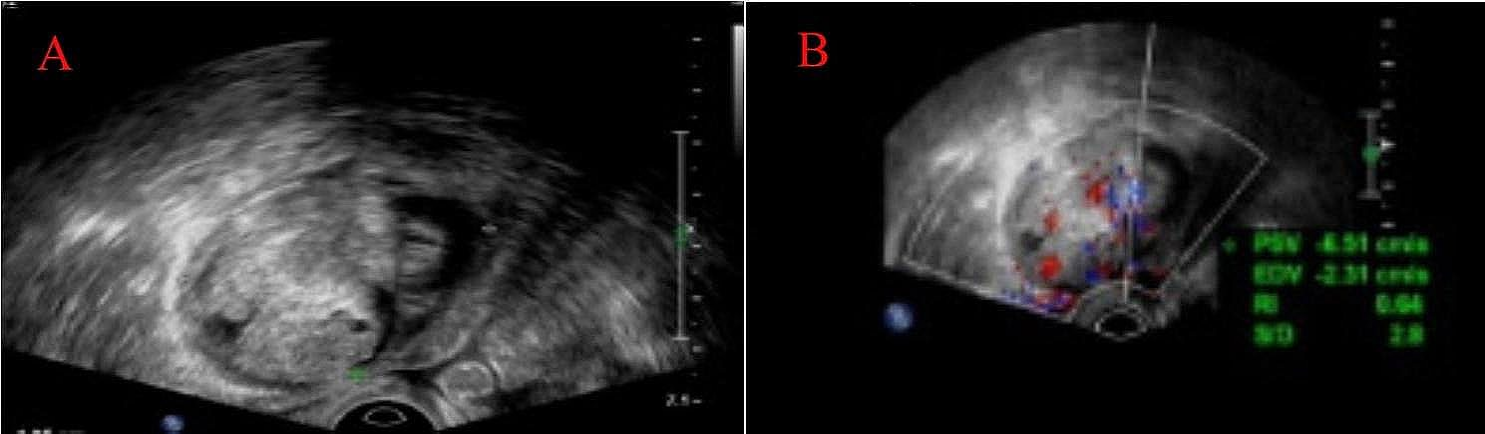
B-ultrasound image. Solid mixed pelvic echo area of 5.0 cm × 3.8 cm × 4.7 cm, with a clear boundary and regular morphology (A). CDFI: Rich blood flow signals can be detected within the arterial spectrum, with the arterial resistive index (RI) at 0.64. A free, nocturnal dark area was found in the pelvic cavity at a depth of about 1.7 cm (B)
